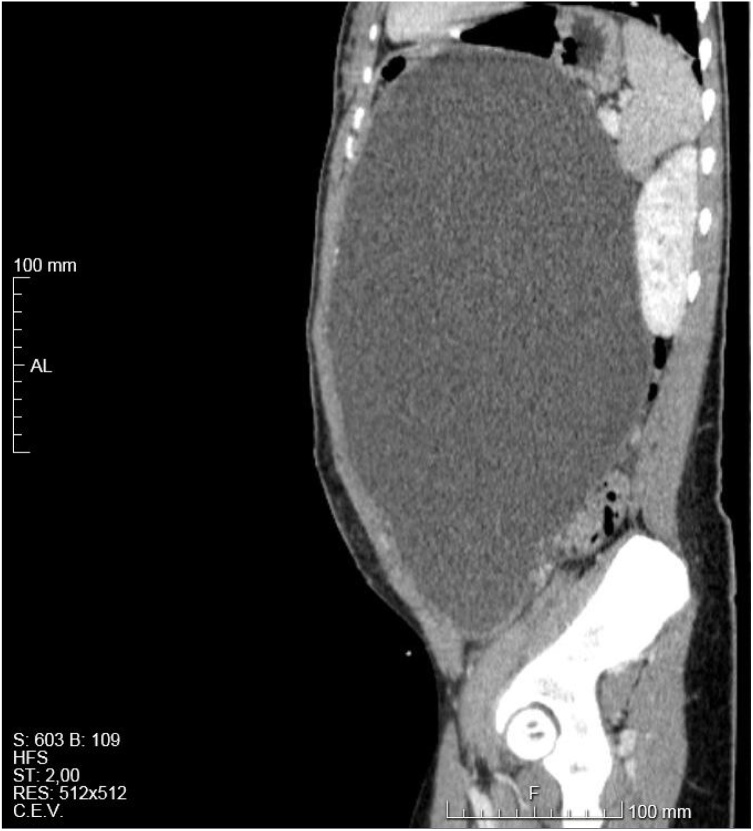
Sagittal image of abdominal and pelvic CT showing the large size of the cyst and its effects on the surrounding structures.
